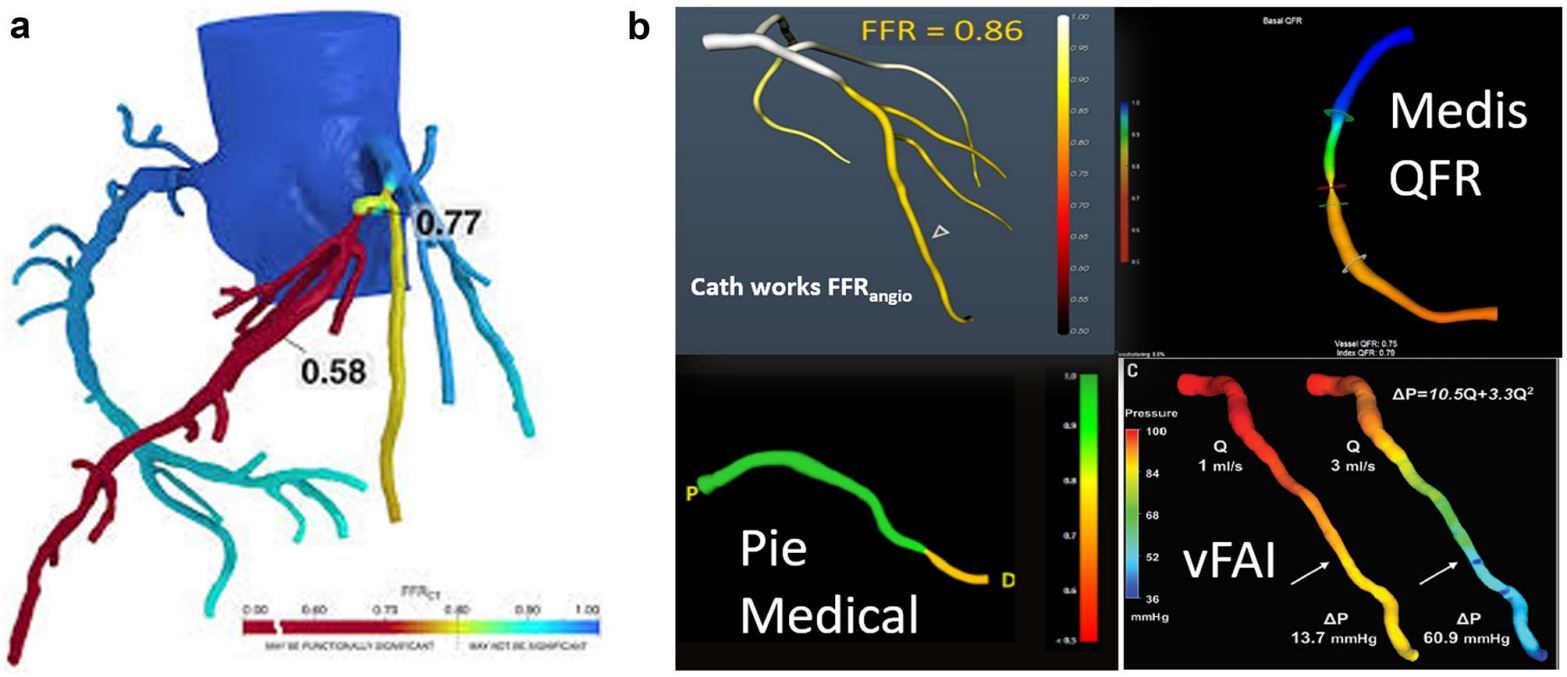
(A) Methodologies for angiographic-derived FFR include CT-based FFR (HeartFlow) prior to invasive physiology, while (B) in-lab approaches include QFR (Medis), FFR_angio_ (CathWorks), CAAS-vFFR (Pie Medical), and vFAI (virtual functional assessment index)
